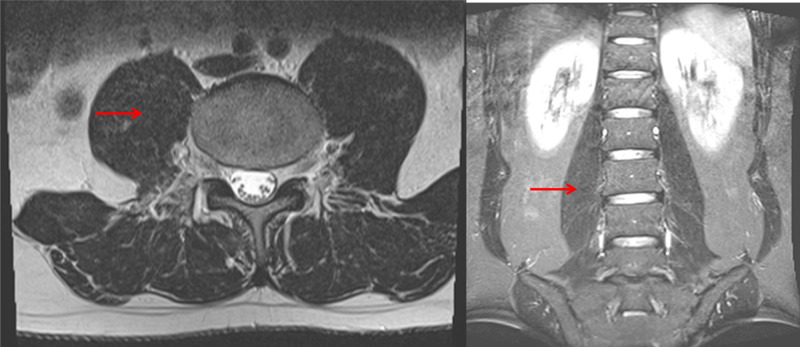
Three months follow-up MRI showing no signs of infection (red arrows): (A) axial plane and (B) coronal plane.
